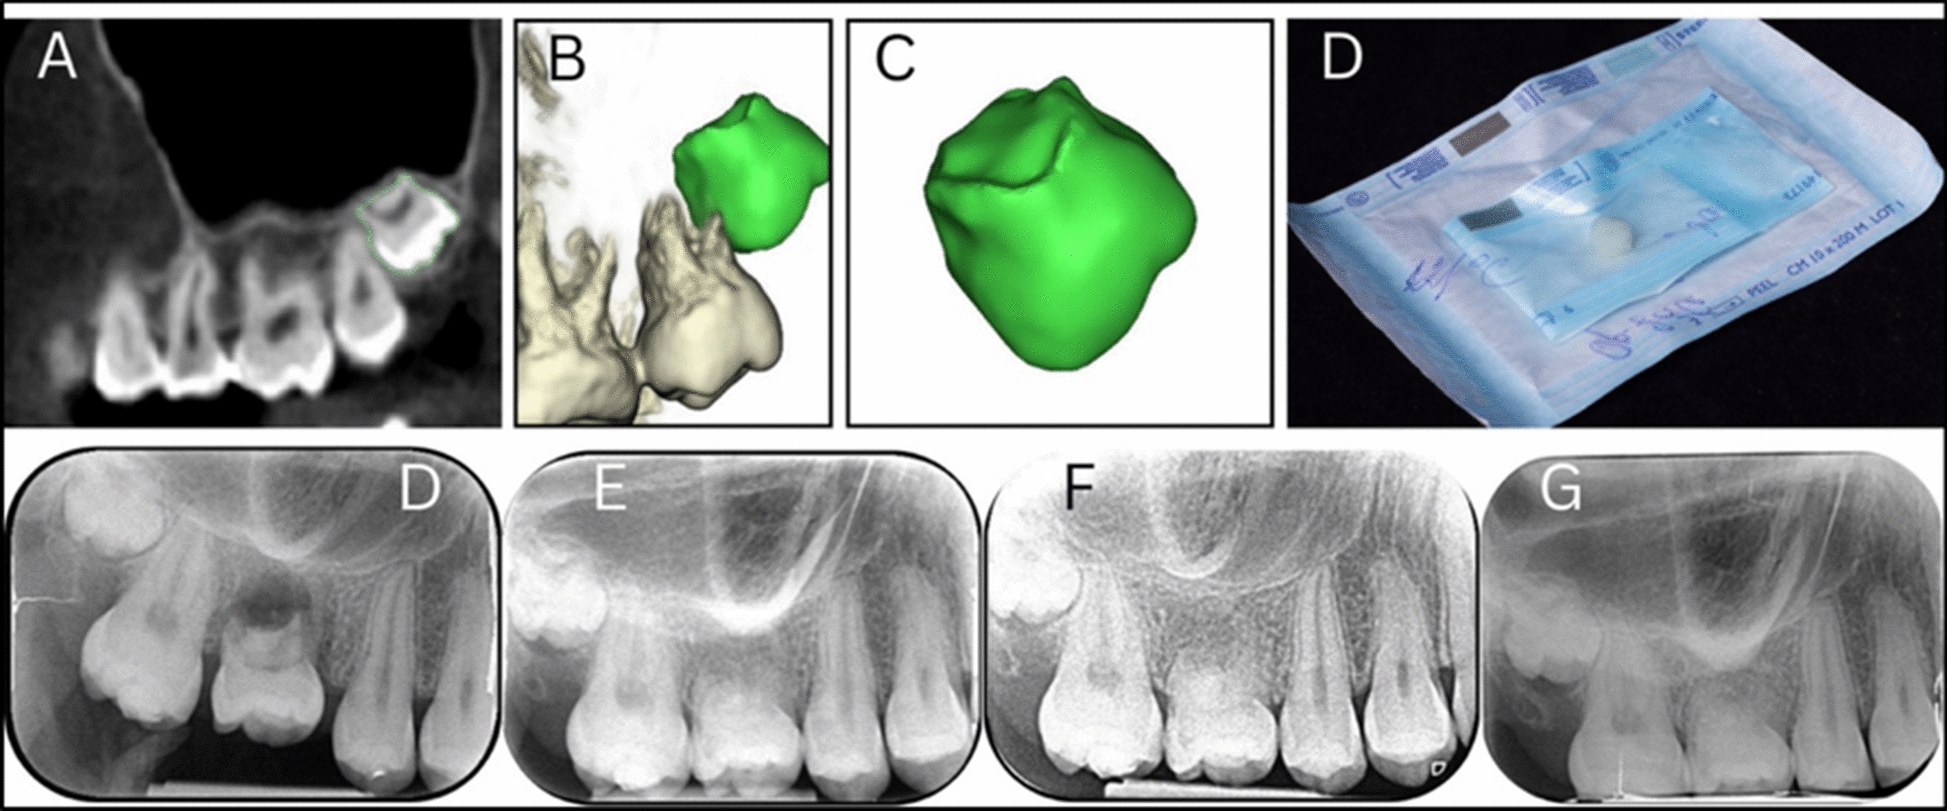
Planning and follow-up of autotransplantation of donor tooth 28 using three-dimensional technology. A Cone-beam computed tomography image showing the donor tooth 28 (outlined in green) selected for autotransplantation. B, C Three-dimensional reconstruction of tooth 28 with surrounding structures and an isolated digital model, used to design a surgical replica. D Sterilized three-dimensionally printed tooth replica packaged for surgical use, enabling trial fitting and minimizing extraoral donor handling. E Periapical radiograph taken immediately after autotransplantation into site 16. F Radiograph at 6-month follow-up, demonstrating early signs of periodontal healing and no signs of resorption or ankylosis. G Follow-up at 1 year showing continued root maturation and periodontal integrity. H Follow-up at 2 years confirming long-term success with stable position; no radiographic signs of pathology
